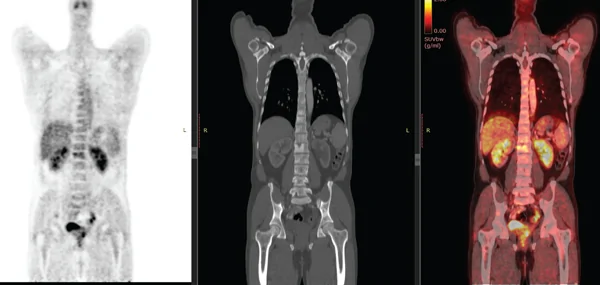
18F‐FDG PET/CT after six cycles of etoposide–cisplatin (May 2025). Prostatic and nodal FDG uptake is markedly reduced compared with baseline. Previously hypermetabolic hepatic and osseous lesions show near‐complete metabolic resolution, indicating a significant partial remission.
